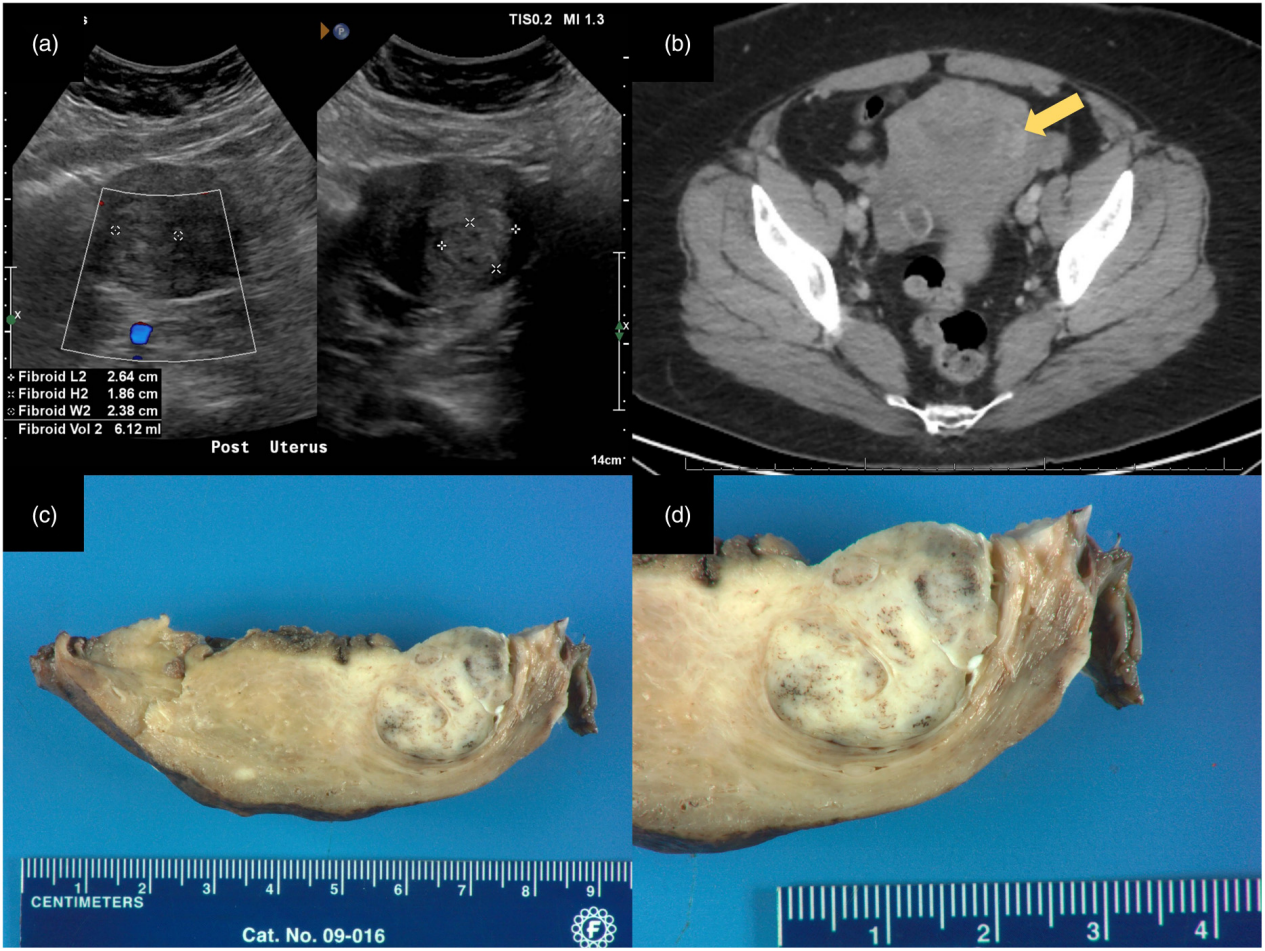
(a) Posterior uterine ultrasonogram showing a hyperechoic lesion in the posterior left uterine wall which measured 2.6 × 1.9 × 2.4 cm^3^; (b) CT abdomen/pelvis with contrast demonstrating enhancing lesion of posterior left uterine wall (yellow arrow; axial view); (c, d) Gross photographs of uterine angioleiomyoma showing a well-circumscribed mixed tan and blue-grey mass at the left posterior lateral myometrium with pinpoint hemorrhages and variegated surface. CT, computed tomography.
